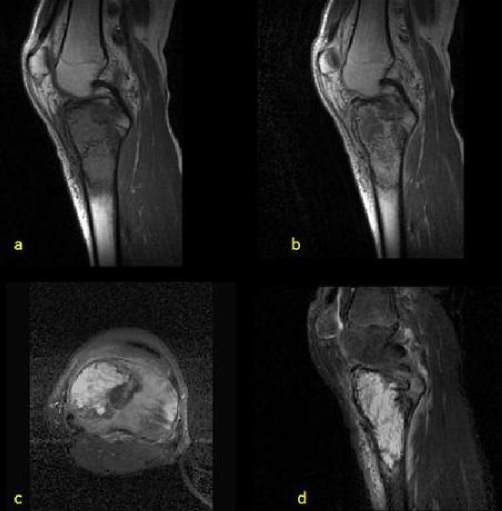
Sagittal T1-weighted image (a), sagittal contrast enhanced T1-weighted image (b), axial (c) and sagittal (d) T2-weighted images reveals a predominantly cystic lesion at the medial aspect of the proximal tibia with septations, fluid-fluid levels and a thick enhancing periphery. Bone marrow edema at the lateral aspect of the tibia and at the subcutaneous tissues also noted.
